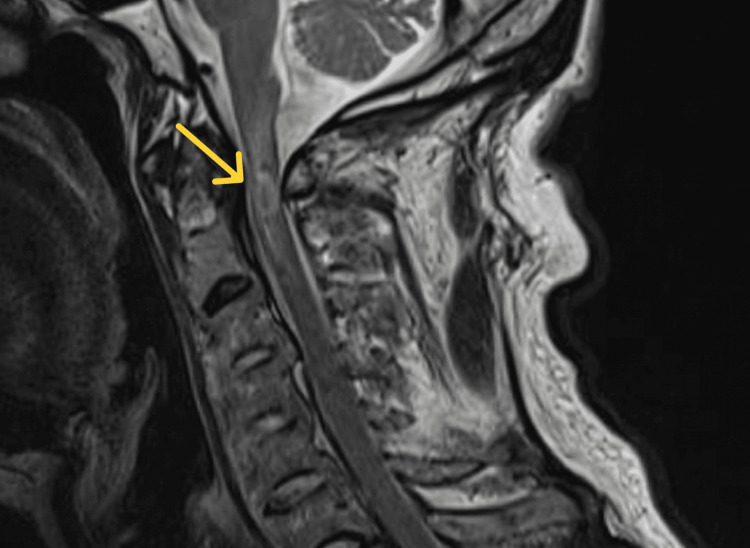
Sagittal T2-weighted MRI of the cervical spine Multilevel spondylotic changes and a diffuse intramedullary T2 hypersignal extending from the bulbomedullary junction to the C3 segment (yellow arrow) can be seen. The most prominent signal changes are observed at the C1-C2 level. Background multilevel disco-osteophytic changes and reduced anterior subarachnoid space are consistent with pre-existing cervical spondylosis.
